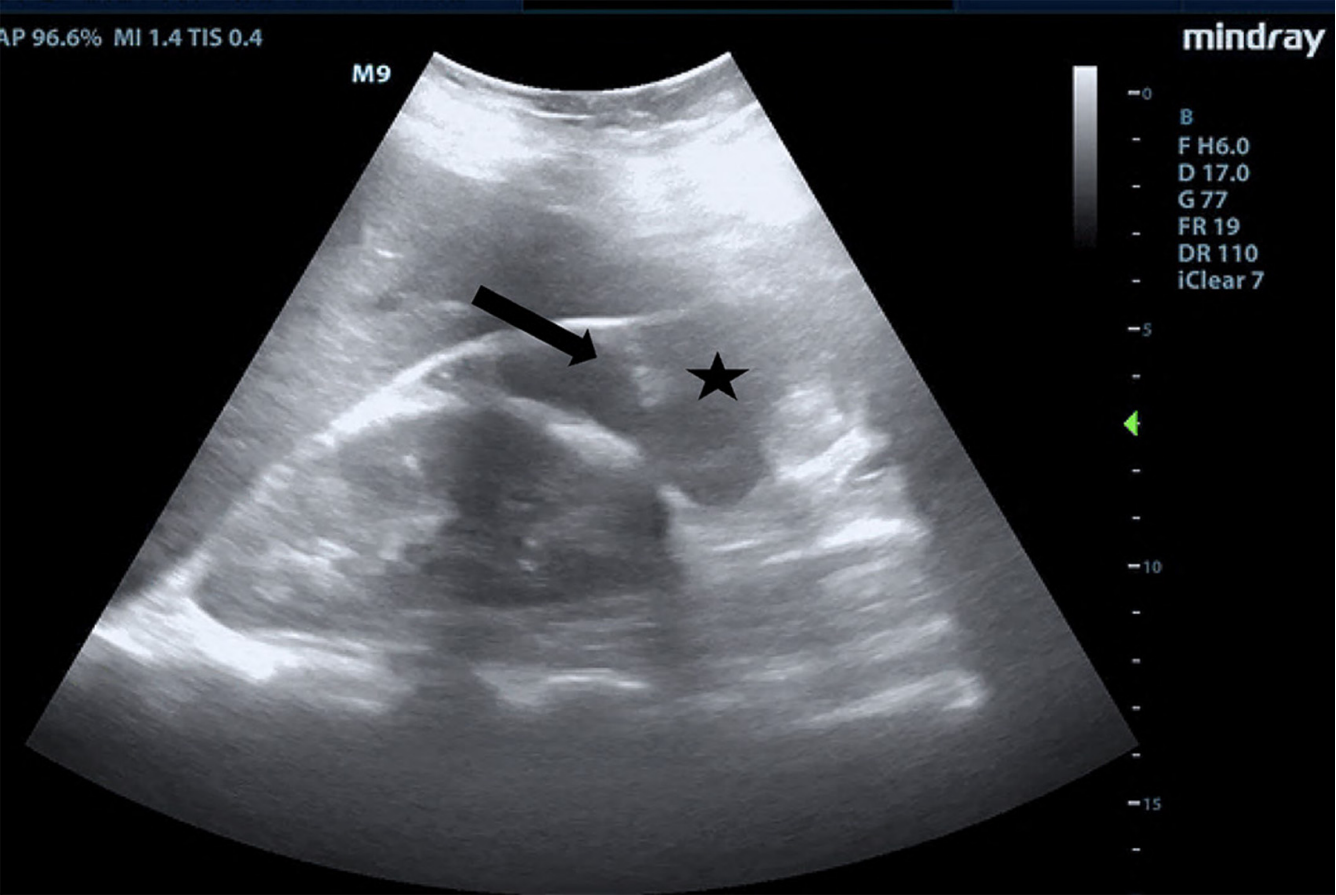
Right upper quadrant ultrasonography using curvilinear probe in the coronal plane demonstrating ascites (black star) and septations (black arrow) in a patient ultimately diagnosed with pelvic inflammatory disease.
